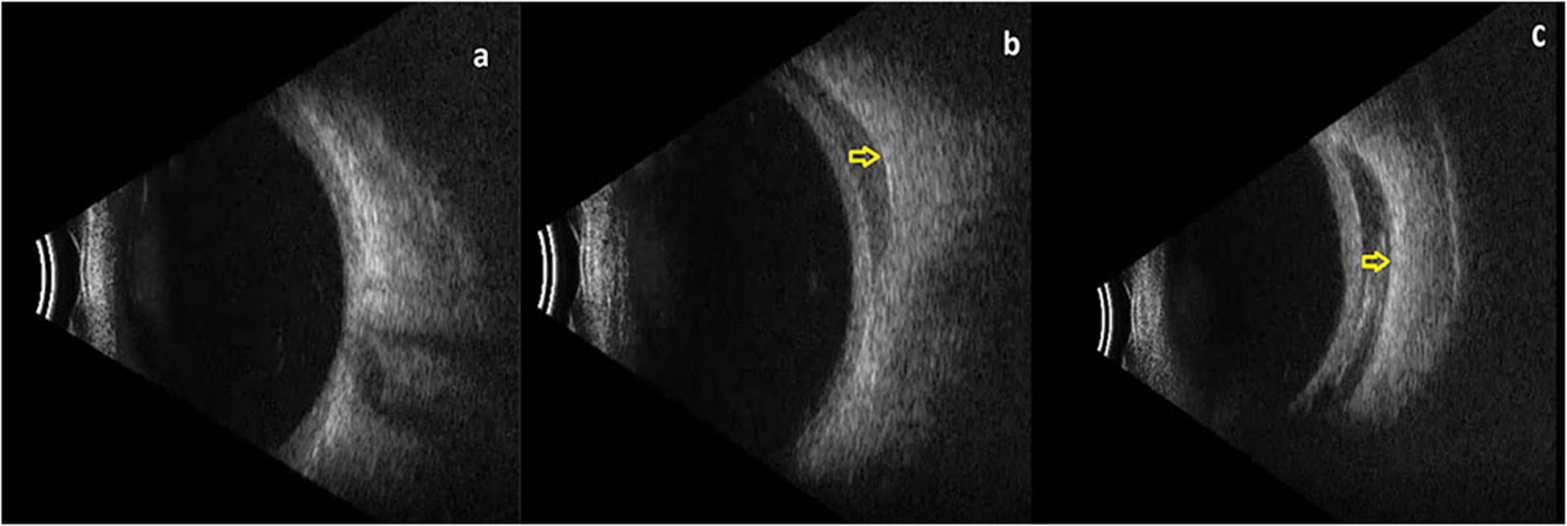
a-c Ultrasonographyic view of WJ-MSC implantation into the deep subretinal space within the extraocular muscle conus; a: before the application (Table 1, patient no. 1), b: injection via 25 G sharp-tip needle (Table 1, patient no. 1), c: placement via 20 G curved subtenon canulla with pre-placed suture to prevent the leakage (Table 1, patient no. 4)
